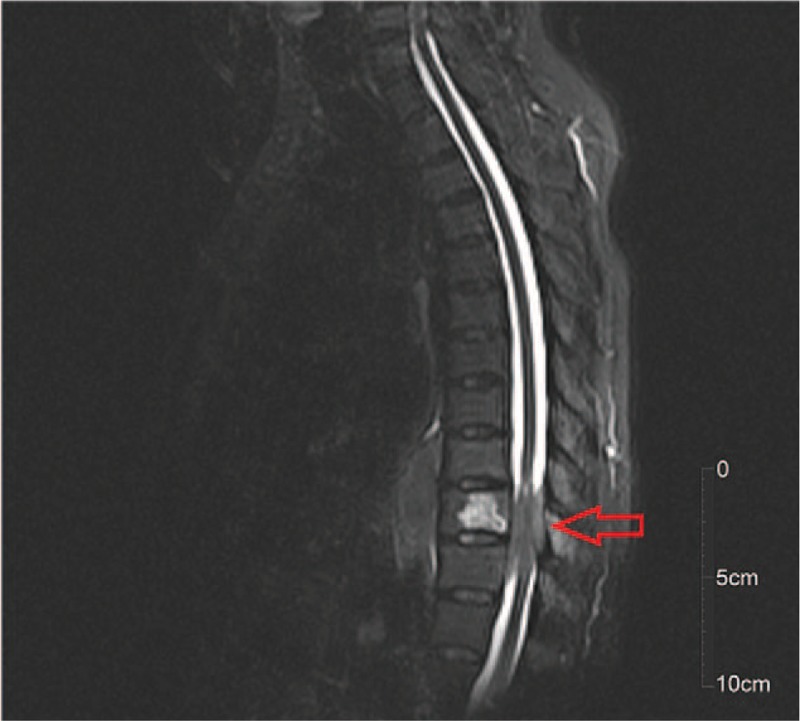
Magnetic resonance imaging (T2-weighted) sagittal images revealed lesions that invaded the spinal canal (red arrow).
